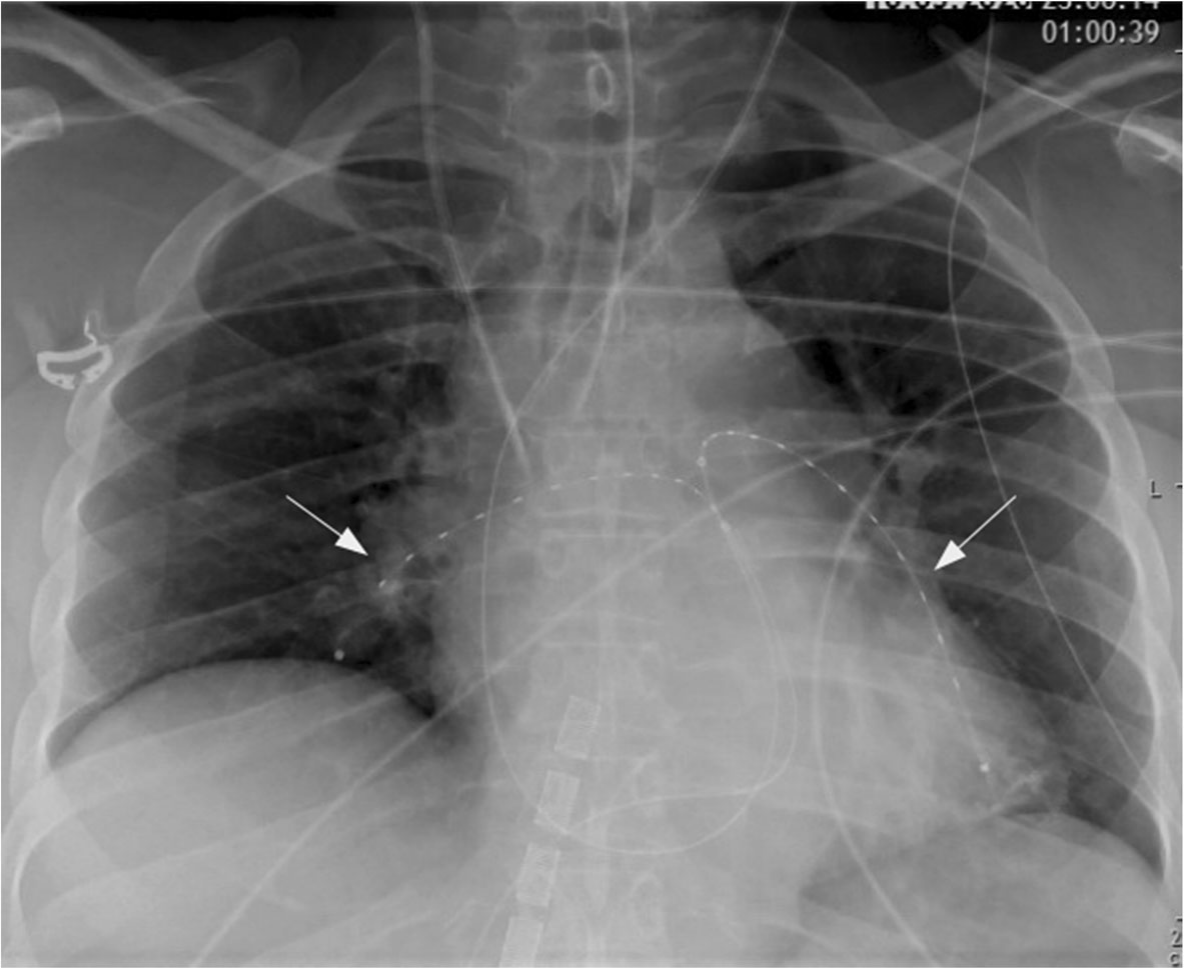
Chest X-ray shows both catheters in situ (arrows)
